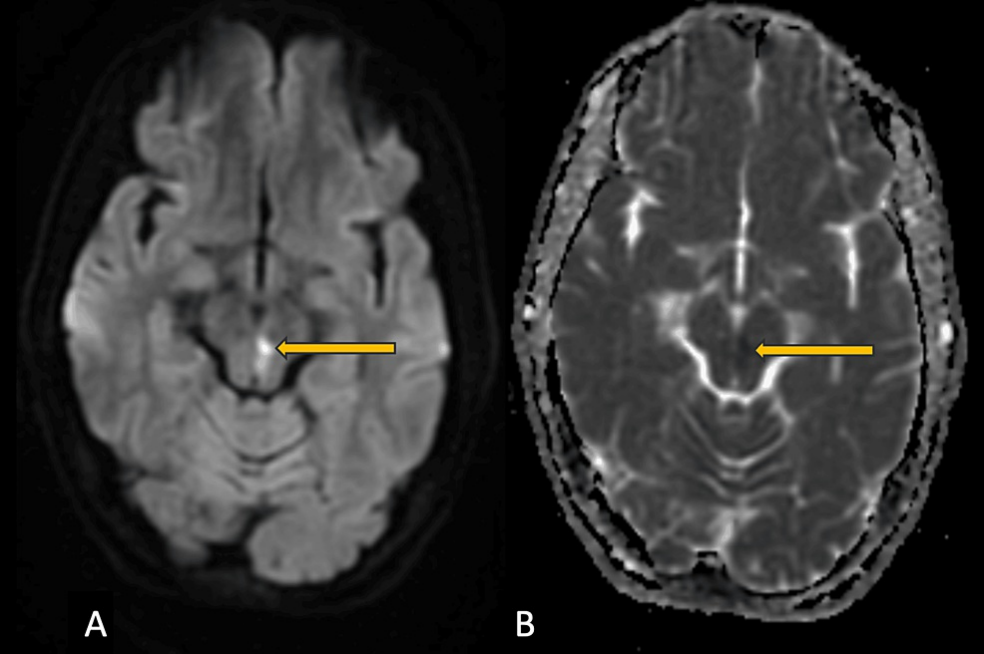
MRI Brain; DWI and ADC images MRI Brain; Diffusion weighted (DW) and apparent diffusion coefficient (ADC) images in the axial plane showing restricted diffusion (yellow arrow) appearing bright on DW image  (A) and corresponding loss of signals on ADC image (B).
